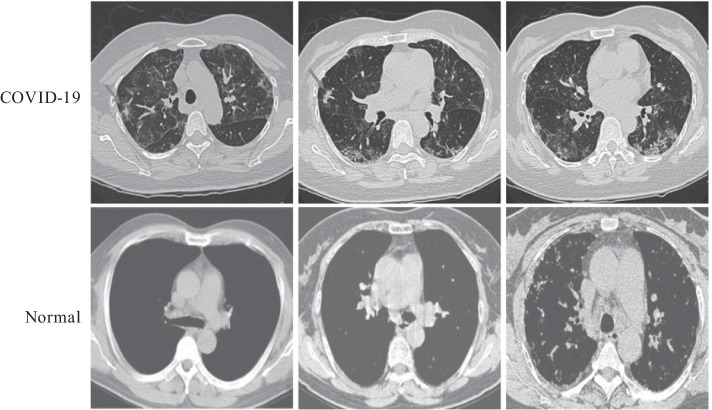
Representative CT images in the COVID-19 and Normal classes
